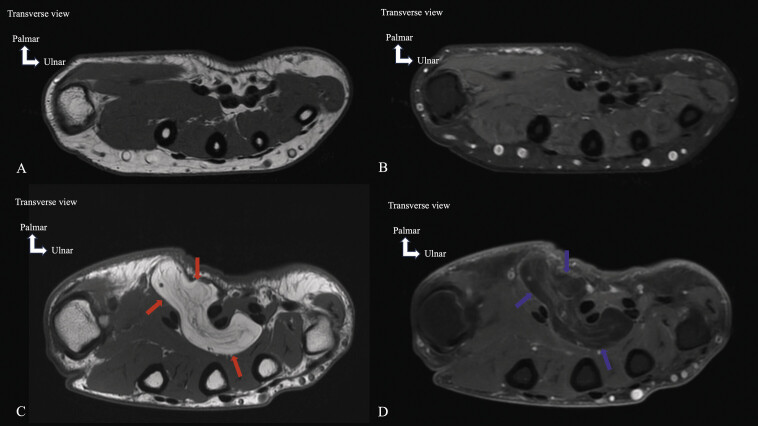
Lipoma. Magnetic resonance imaging in axial T1-weighted (
